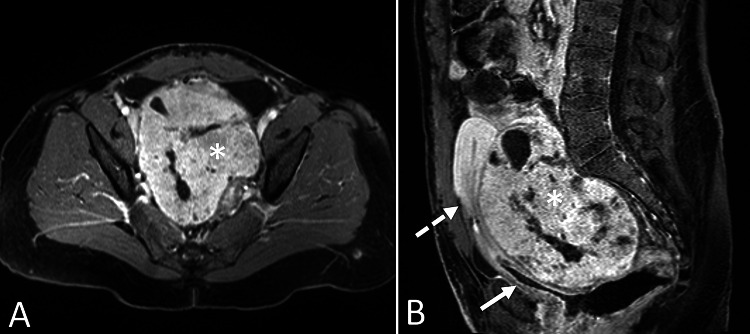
Post-contrast pelvic MRI. T1 weighted post-contrast pelvic MRI in axial (A) and sagittal (B) planes demonstrates a large, predominantly solid, and avidly enhancing mass (asterisks) occupying most of the pelvis. There is associated mass effect on the bladder (solid arrow) and uterus (dashed arrow).
